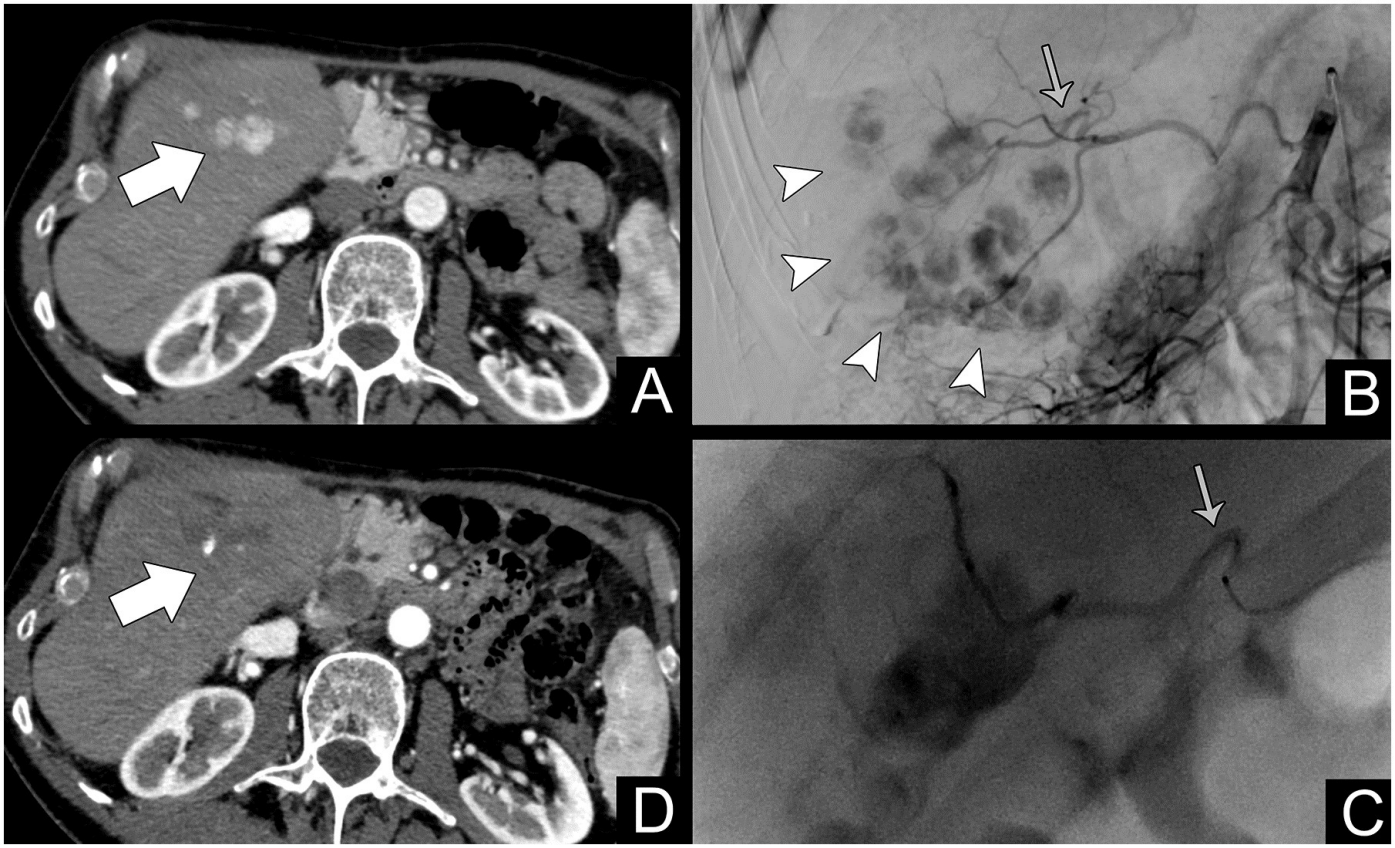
Patient #267, treated for multifocal HCC at 72 years (BCLC B). A: Contrast-enhanced CT arterial phase showing a multifocal hypervascular HCC in the fifth segment (white arrow); B: Selective angiography of the right hepatic artery showing the extent of the disease (white arrowheads). The gray arrow points to the main feeding vessel; C: Superselective catheterization (gray arrow) and embolization of the main feeding vessel (with *G*-TAE); D: One-month follow-up showing complete devascularization with a small hyperdense spot representing residual Lipiodol (white arrow). The patient was still alive at the end of the study with a survival of 17.4 months.
